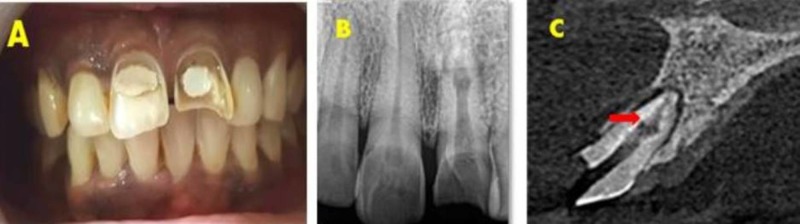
Preoperative assessment for patient with inflammatory non-perforating internal root resorption. (A) Pre-operative photograph. (B) Pre-operative radiograph. (C) CBCT image showing sagittal view of tooth 21 with an internal resorptive defect. CBCT: Cone beam computed tomography
